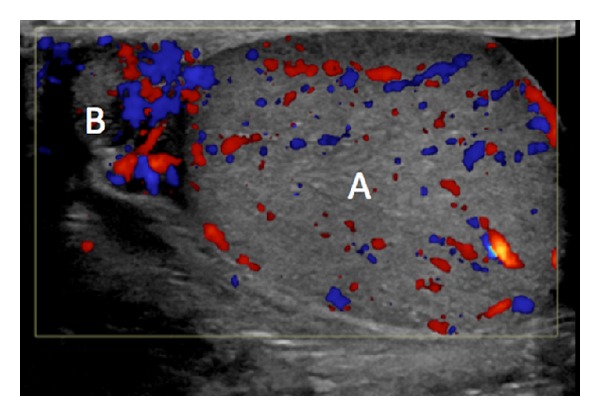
Right scrotal ultrasound with color Doppler interrogation at presentation to the ED showing A: right testis with preserved vascularity; B: right epididymis with enlargement and slightly increased vascularity.
